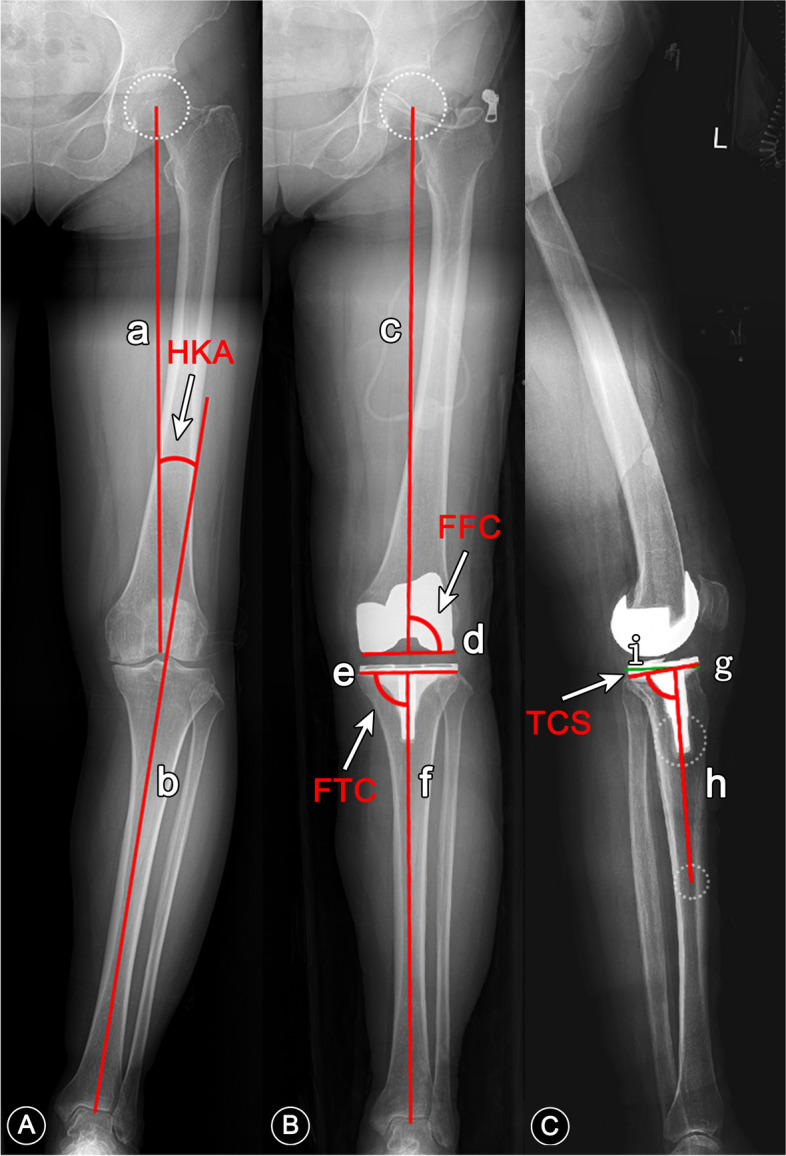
Measurements of HKA angle, FFC, FTC and TCS. HKA: hip-knee-ankle; FFC: frontal femoral component angle; FTC: frontal tibial component angle; TCS: tibial component slope angle; A Preoperative HKA angle: the acute angle formed between the femoral mechanical axis (line a) and the tibial mechanical axis (line b). B Line c: postoperative femoral mechanical axis; line d: the line across the bottom of the femoral condyles; line e: the line across the bottom of the tibial plateau on the anteroposterior radiograph; line f: the postoperative tibial mechanical axis; FFC: the lateral angle between line c and line d; FTC: the medial angle between line e and line f; postoperative HKA angle: the acute angle between line c and line f. C Line g: the line across the bottom of the tibial plateau on the lateral radiograph; line h: the line connecting the center points of the tibial shaft 5 cm and 15 cm below the joint line; line i is perpendicular to line h; TCS: the acute angle between line i and line g
